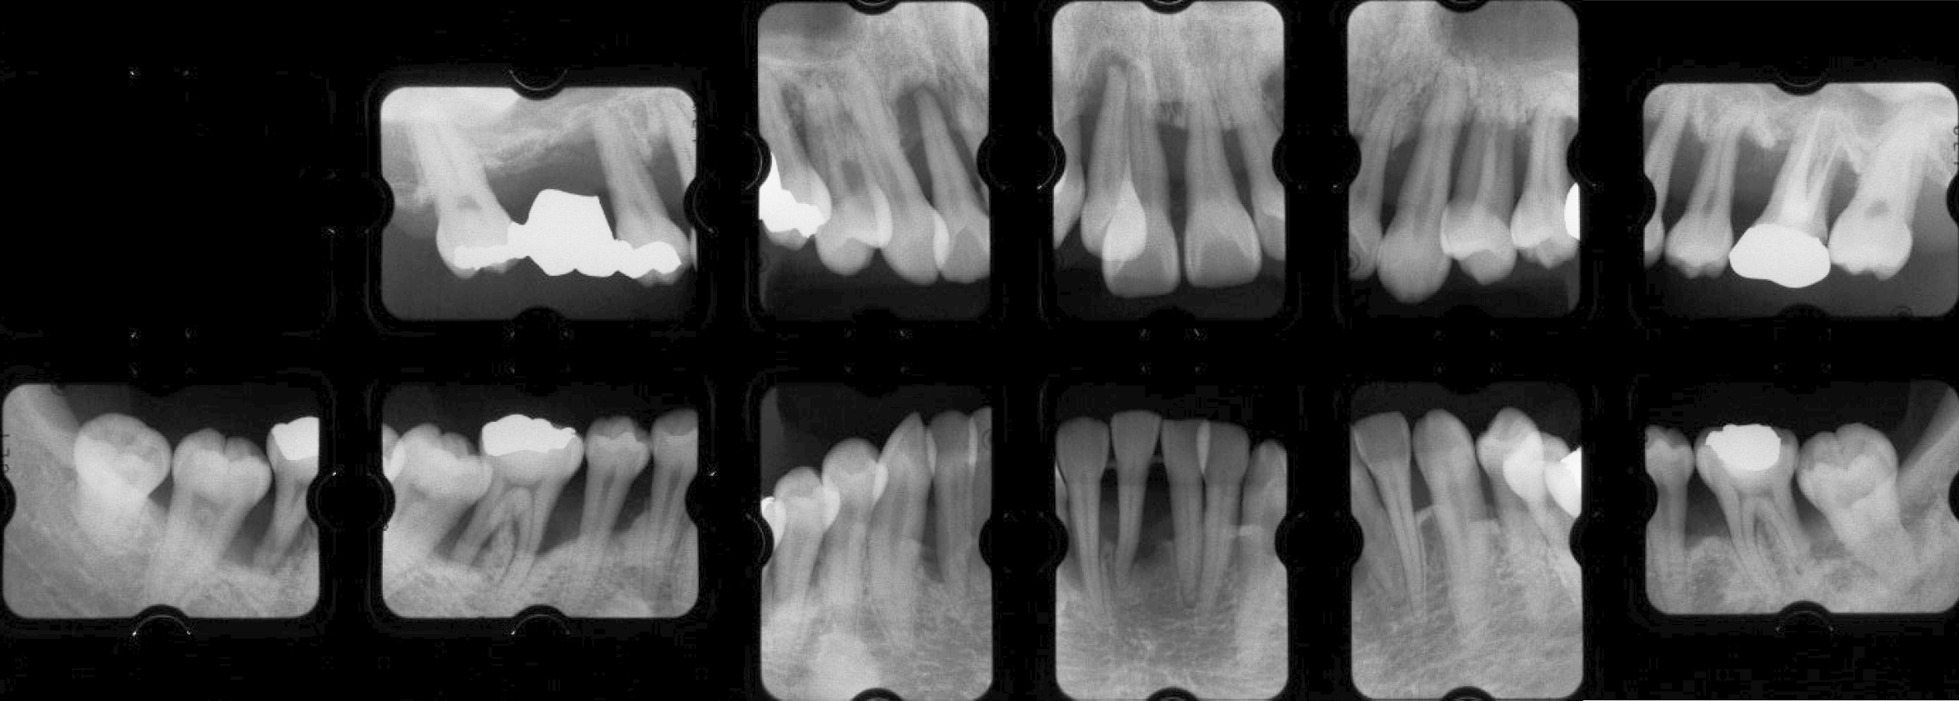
Dental periapical radiography reveals generalized horizontal and vertical bone loss
